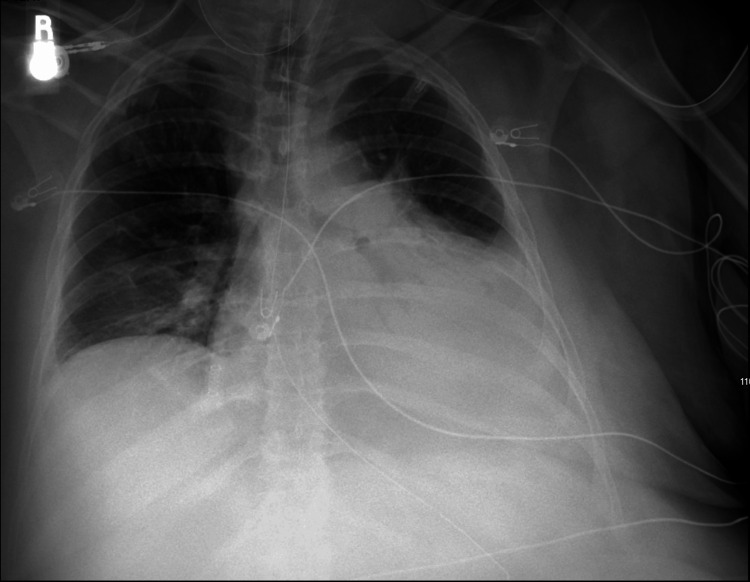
Initial chest X-ray imaging significant for bilateral pleural effusions and lower lobe infiltrates Portable chest radiograph obtained in the anteroposterior (AP) view.
